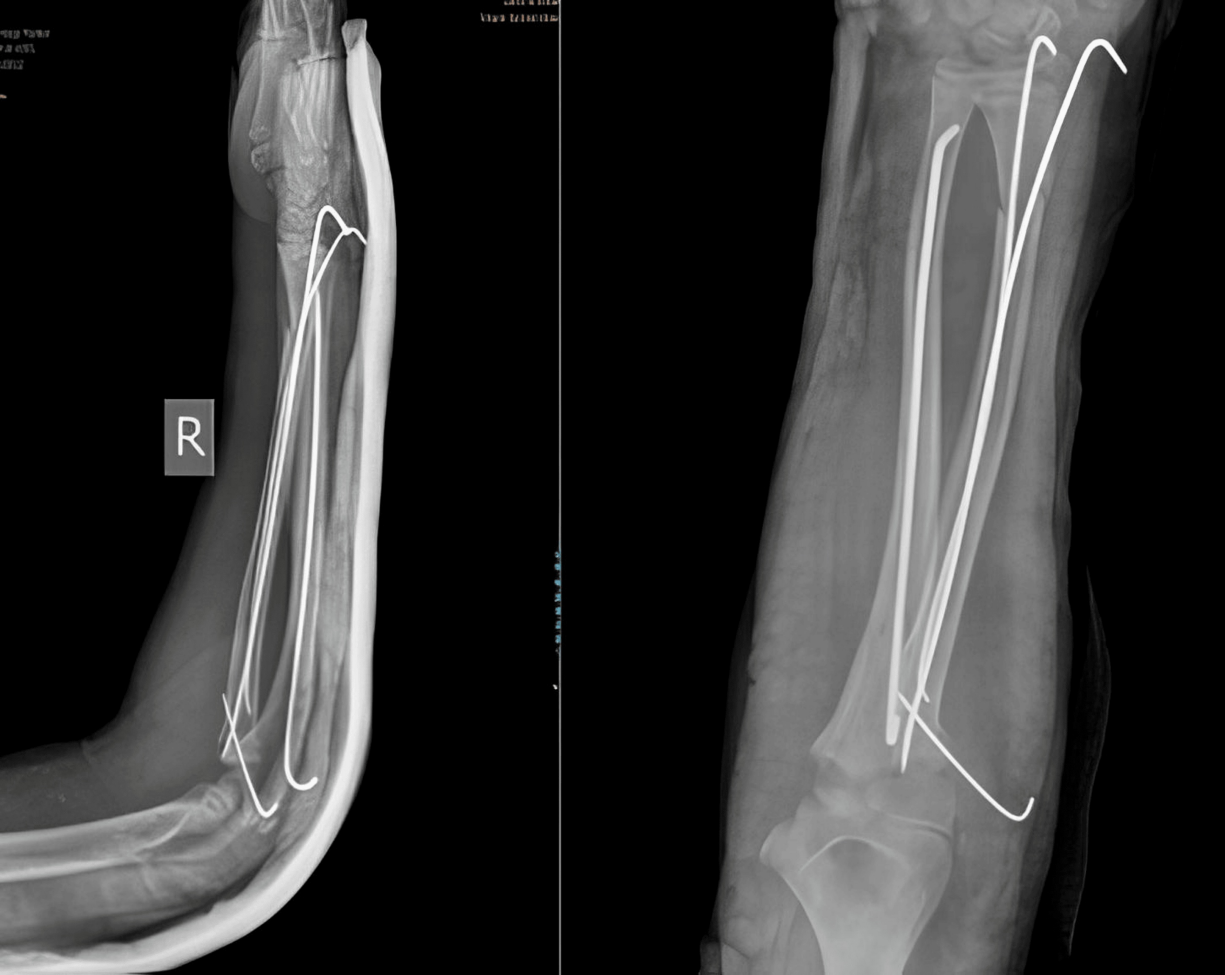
Postoperative X-ray of case 1
